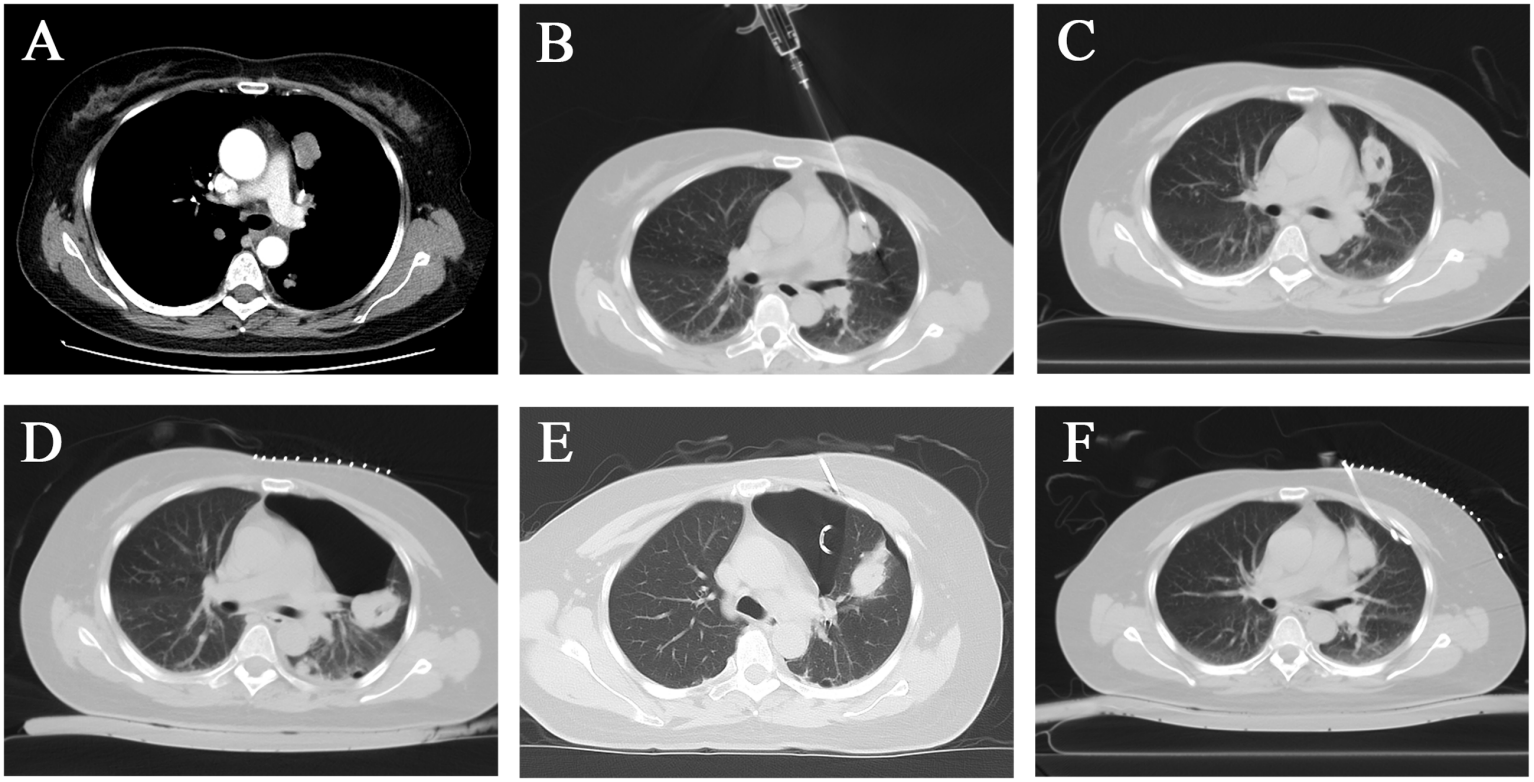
After CT-guided percutaneous biopsy, the patient developed pneumothorax and decreased oxygen saturation, which was treated with CT-guided tube drainage. (A) A left upper lobe mass with significant enhancement. (B) The needle slot of the 18G semi-automatic puncture needle was located in the center of the tumor. (C) No significant postoperative bleeding or pneumothorax was observed. (D) Pneumothorax was caused by the harsh coughing of the patient. (E) The patient was treated with a chest tube for drainage. (F) The pneumothorax had utterly disappeared after 1 week.
